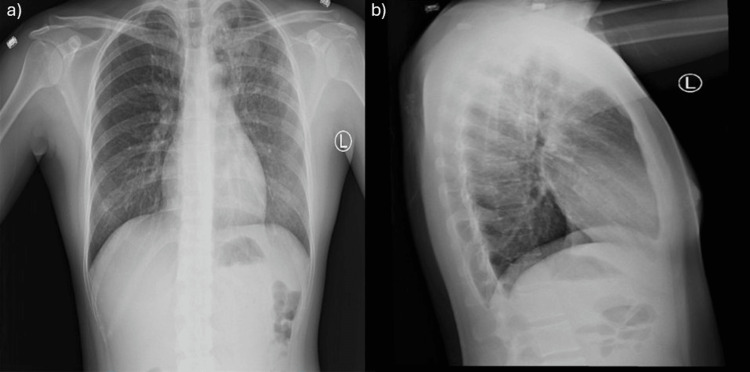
Chest X-ray. (a) Posteroanterior view demonstrating hazy opacification of the left upper lobe with air bronchograms and multifocal reticulonodular opacities, consistent with an infectious or inflammatory pulmonary process. (b) Lateral view confirming left upper lobe consolidation consistent with an infectious or inflammatory process such as pulmonary tuberculosis.
